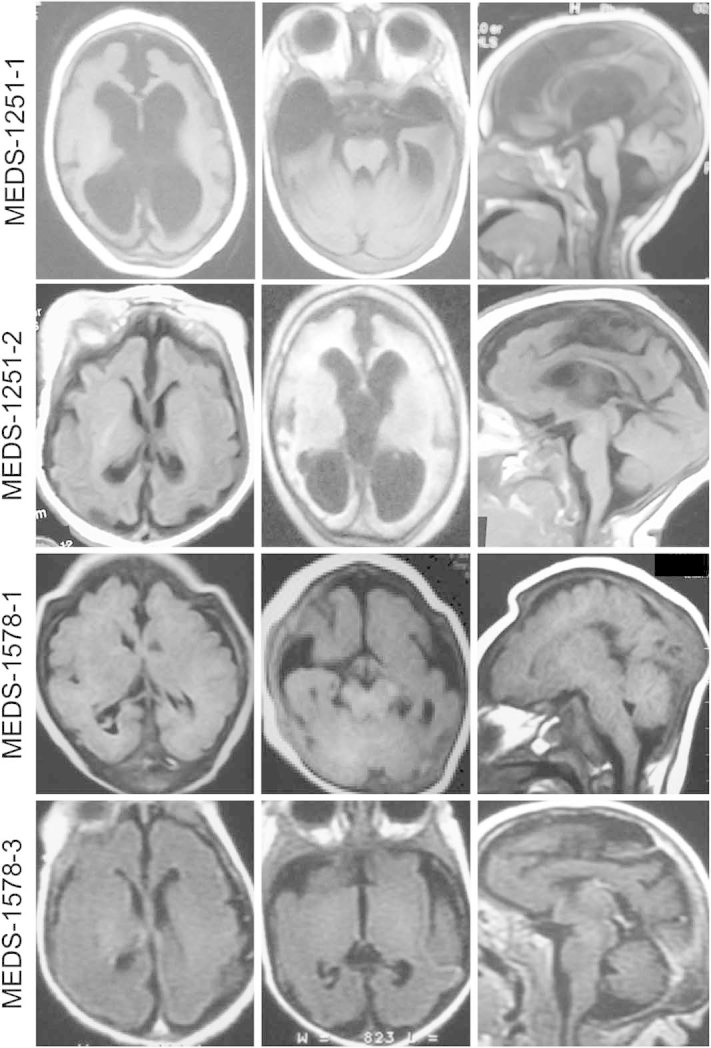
Cranial MRI of patients. First column: Rostral axial T1 weighted images show simplified gyral pattern, severe microcephaly, and different degree of ventriculomegaly, suggestive for cerebral atrophy. Second column: Caudal axial T1 weighted images showing brain atrophy and simplified gyral pattern. Third column: Sagittal images showing severely reduced volume of corpus callosum and cerebellar vermis hypoplasia.
